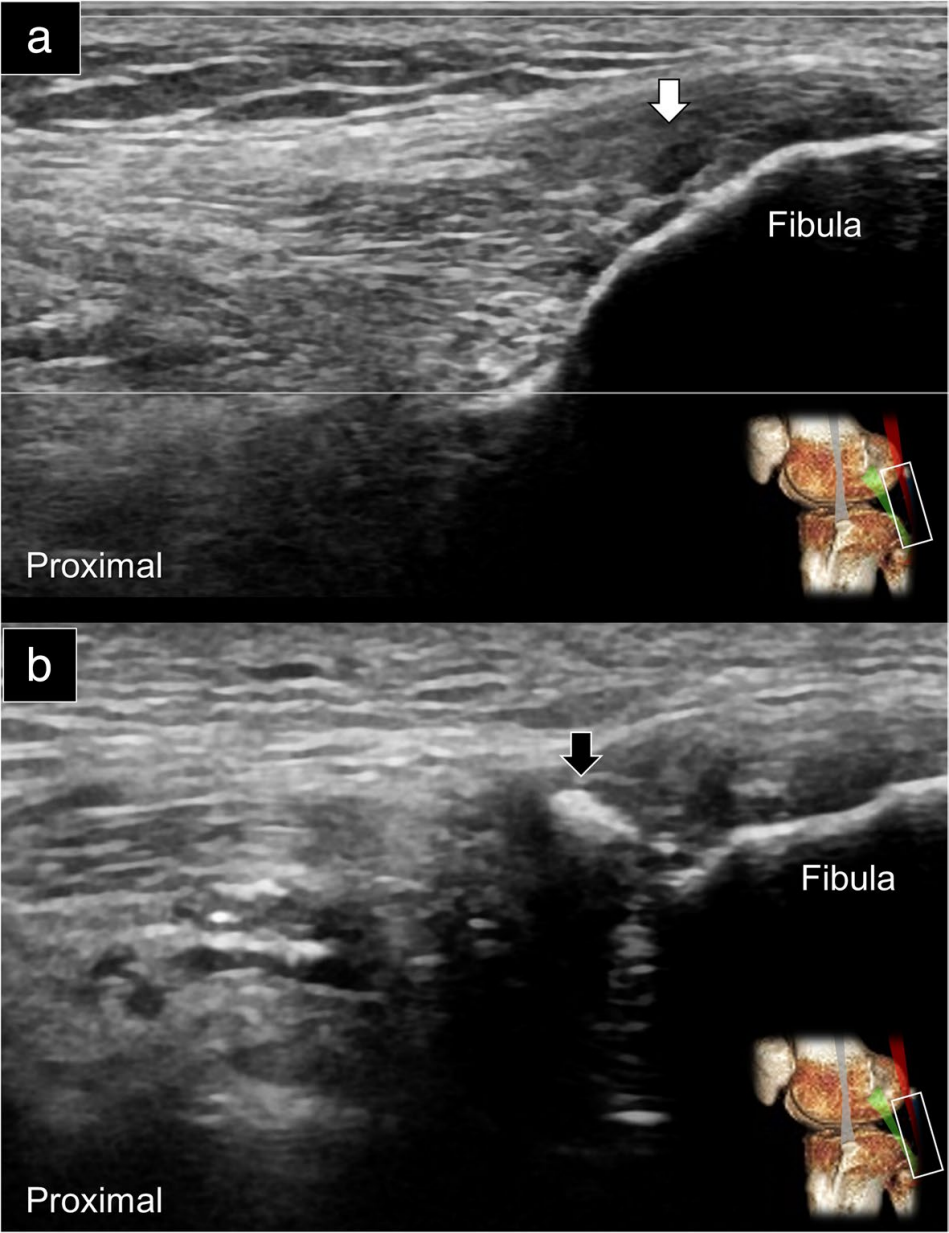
Ultrasound imaging using the long-axis approach revealed an anechoic region, which was consistent with a partial thickness tear (white arrow) of the biceps femoris tendon (a) and calcification inside its distal insertion (black arrow) (b). Please also reference Supplemental Fig. 2 for the normal appearance of the biceps femoris tendon
